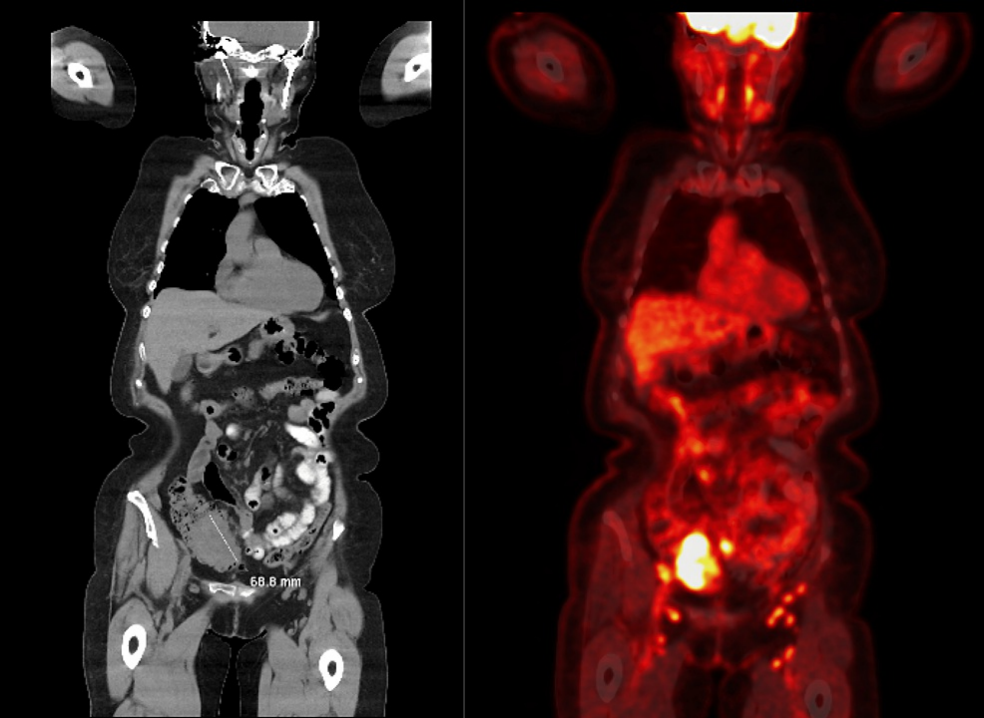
A coronal view CT scan displayed a cecal mass measuring approximately 7 cm with hyper-metabolic activity (left panel); positron emission tomography (PET) scans revealed extensive hyper-metabolic adenopathy extending from the abdomen to the neck, including a 1.8 cm lymph node in the right lower quadrant mesenteric region and peri-pancreatic area (right panel).
